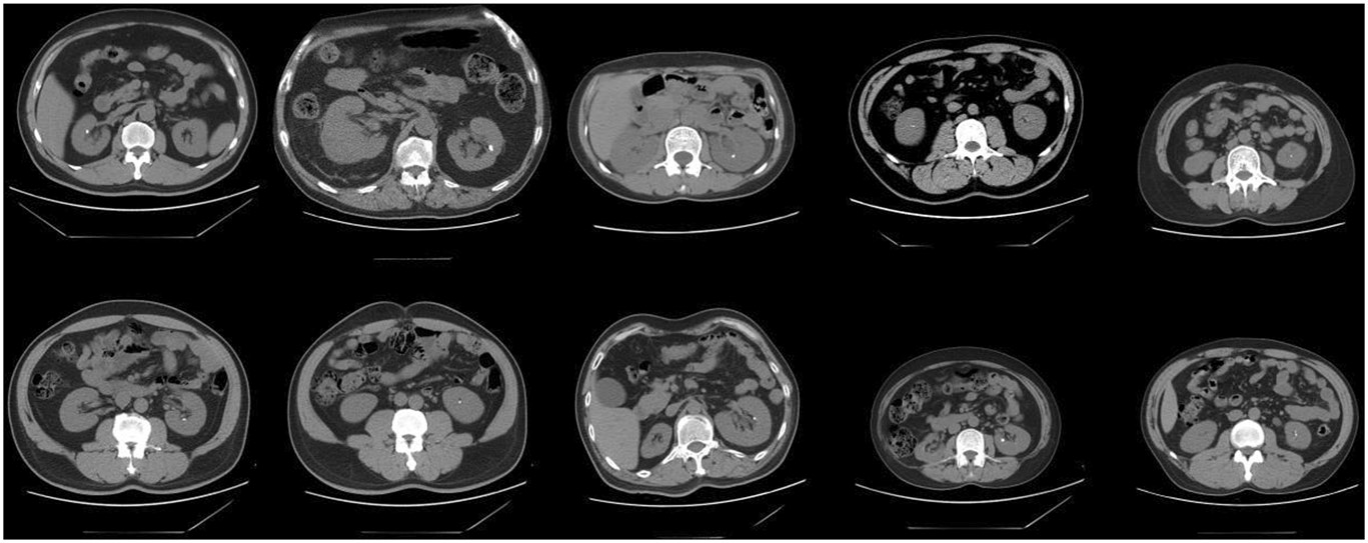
CT images of renal calculi in 10 donor kidneys.
